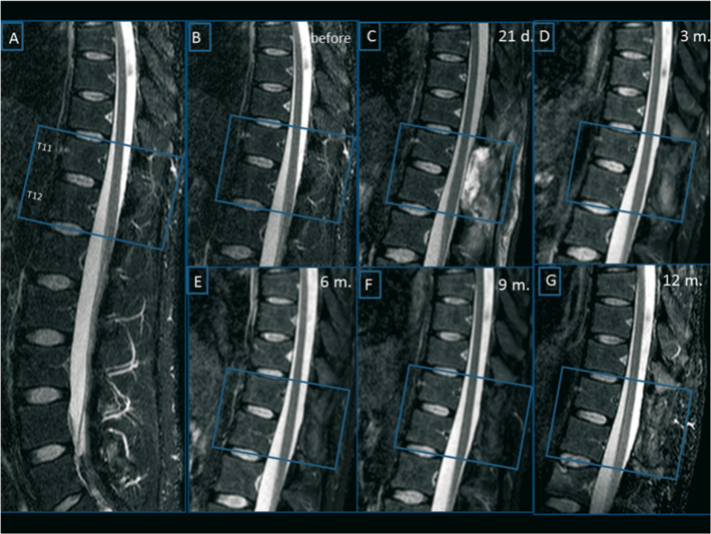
MRI Follow-up. T2 weighted sequences acquired on sagittal plane before surgery (images A-B) and respectively 21 days (image C), 3 (image D), 6 (image E), 9 (image F) and 12 months (image G) after transplantation. Post-surgical MR scans revealed an expected extradural fluid collection at the site of surgery, which resolved spontaneously. No structural changes were detected after hNSCs transplantation relative to the baseline.
